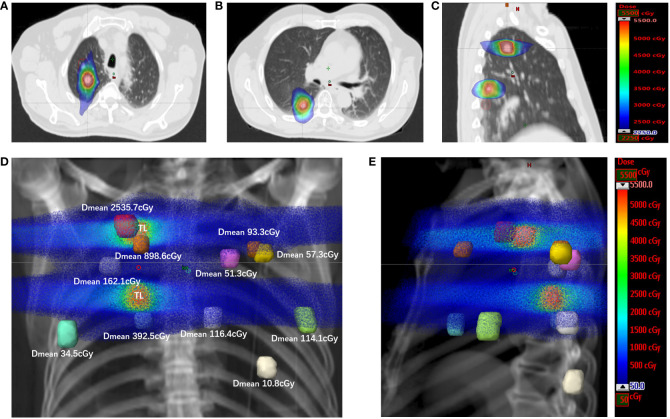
The spatial dose distribution for target and nontarget metastases of the SBRT plan. (A) The dose distribution for the target metastasis located in the right upper lung, with displayed isodoses ranging from 2250 cGy to 5500 cGy. (B) The dose distribution for the target metastasis located in the right lower lung, with the displayed isodoses ranging from 2250 cGy to 5500 cGy. (C) The dose distributions for target metastases in sagittal sections, with the displayed isodoses ranging from 2250 cGy to 5500 cGy. (D) The spatial dose distribution for target and nontarget metastases in the DDR coronal plane. Each colored nodule represents the planning volume of different target or nontarget metastases (uniformly expanded 5 mm for metastases), and the mean dose for the planning volume of each nontarget metastasis is displayed on the side (D_mean_). The displayed isodoses ranged from 50 cGy to 5500 cGy. TL, target lesion; DDR, direct digital radiography. (E) The spatial dose distribution for target and nontarget metastases in the DDR sagittal plane. Each colored nodule represents the planning volume of different target or nontarget metastases. The displayed isodoses ranged from 50 cGy to 5500 cGy. TL, target lesion; DDR, direct digital radiography.
